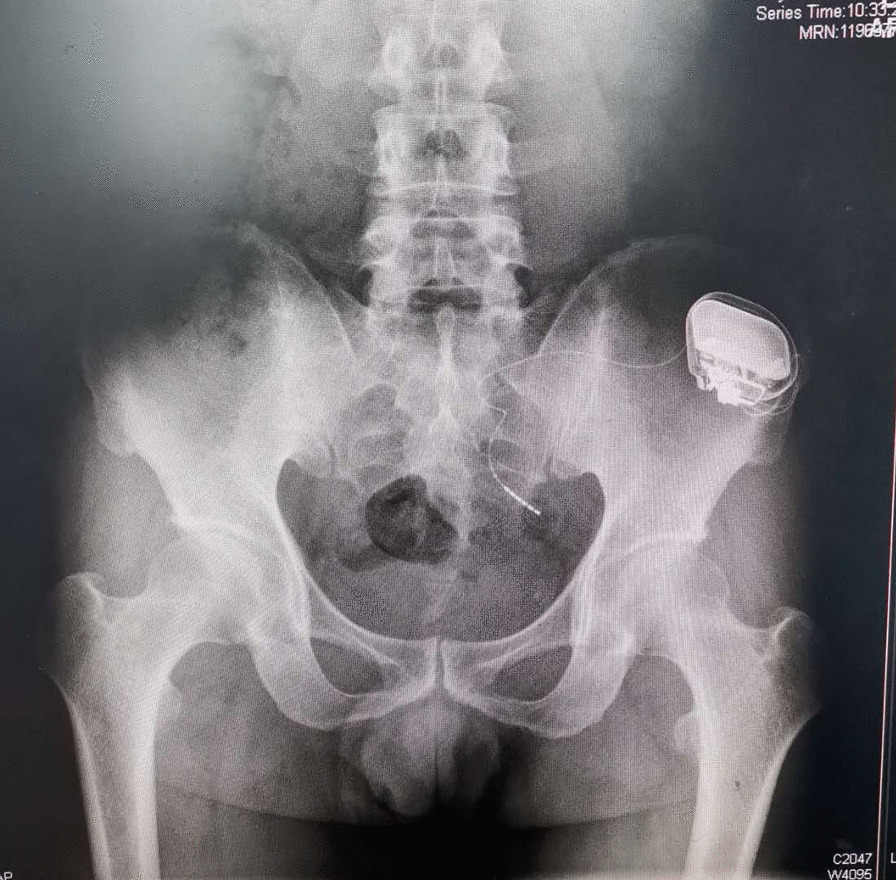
The radiograph of the implantable pulse generator in the patient’s left buttock
